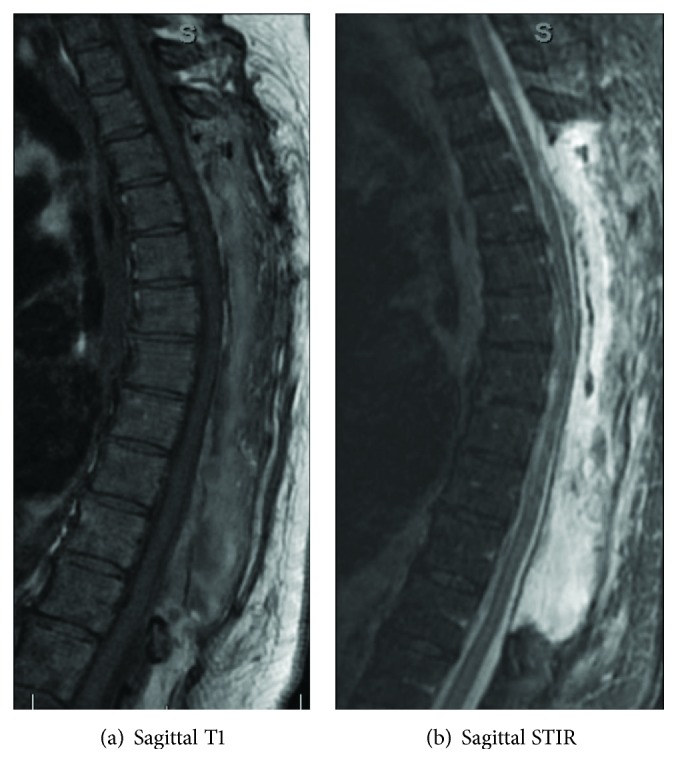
Sagittal T1 (a) and STIR (b) postoperative MRI images of the thoracolumbar spine showing a large multilevel thoracolumbar laminectomy. Postoperative fluid collections are demonstrated on the STIR image.
